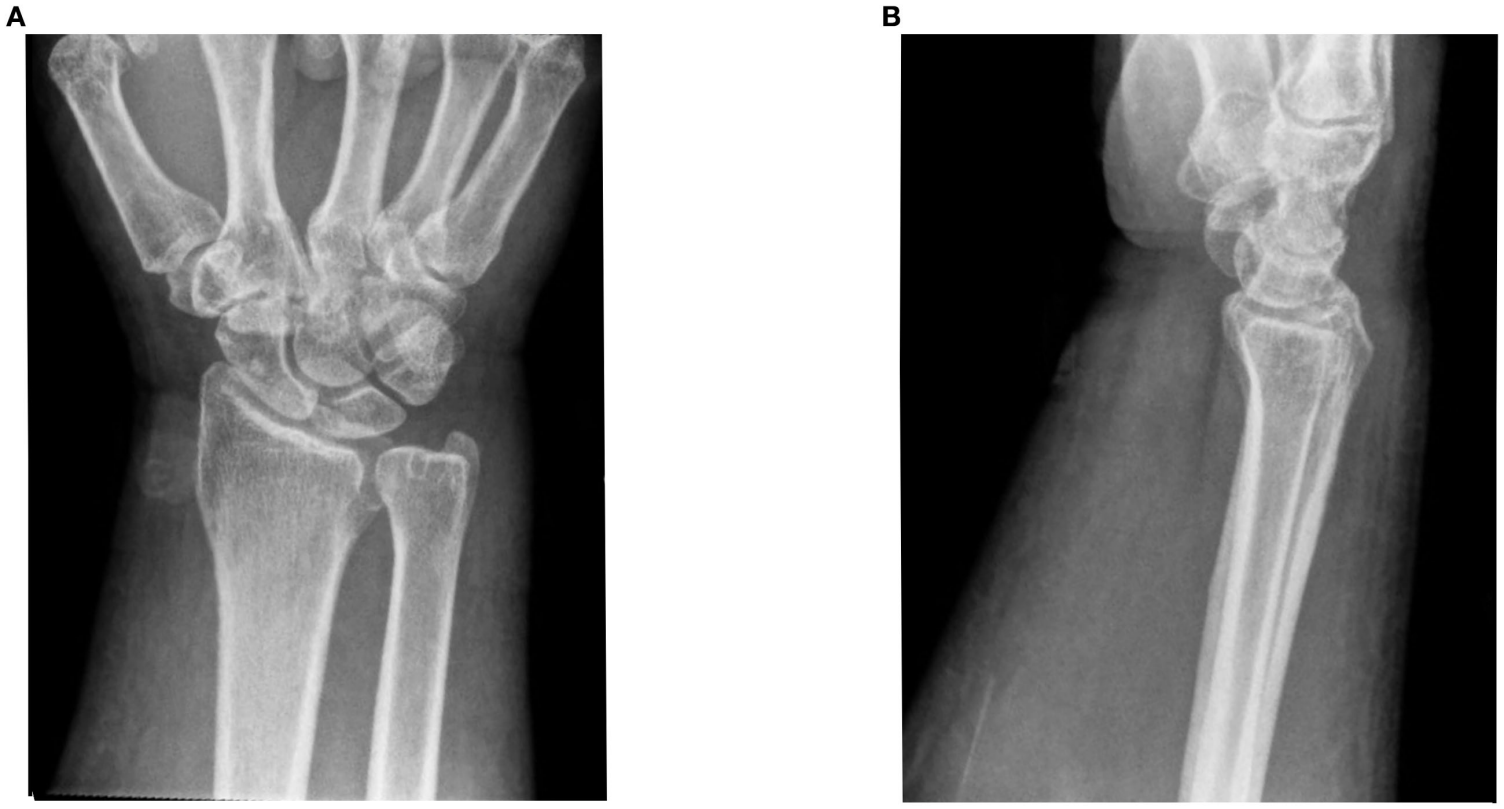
Right wrist x-rays. (A) DA and lateral filme of right wrist ob Im (B) Lateral film diffuse soft tissue inflammation.
